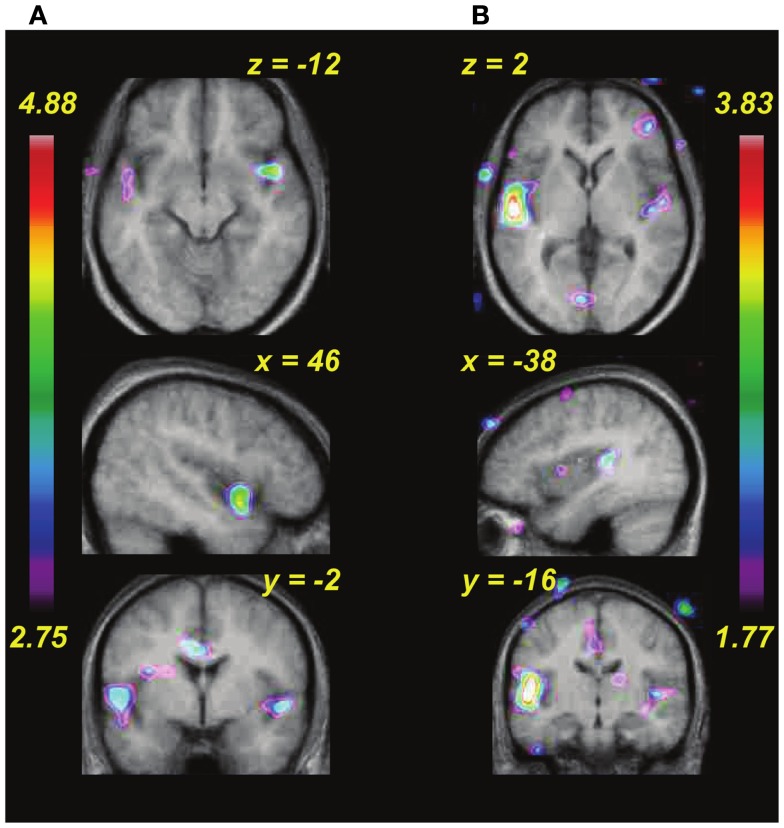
Functional MRI data showing changes in covariation of BOLD activity with increasing pitch interval size during pre-training as a function of the individual rate of learning during training (i.e., behavioral data shown in Figure 4). (A) Passive task. (B) Active task. In both tasks, auditory cortical areas respond more to increasing pitch interval size in those individuals who subsequently showed faster learning.
